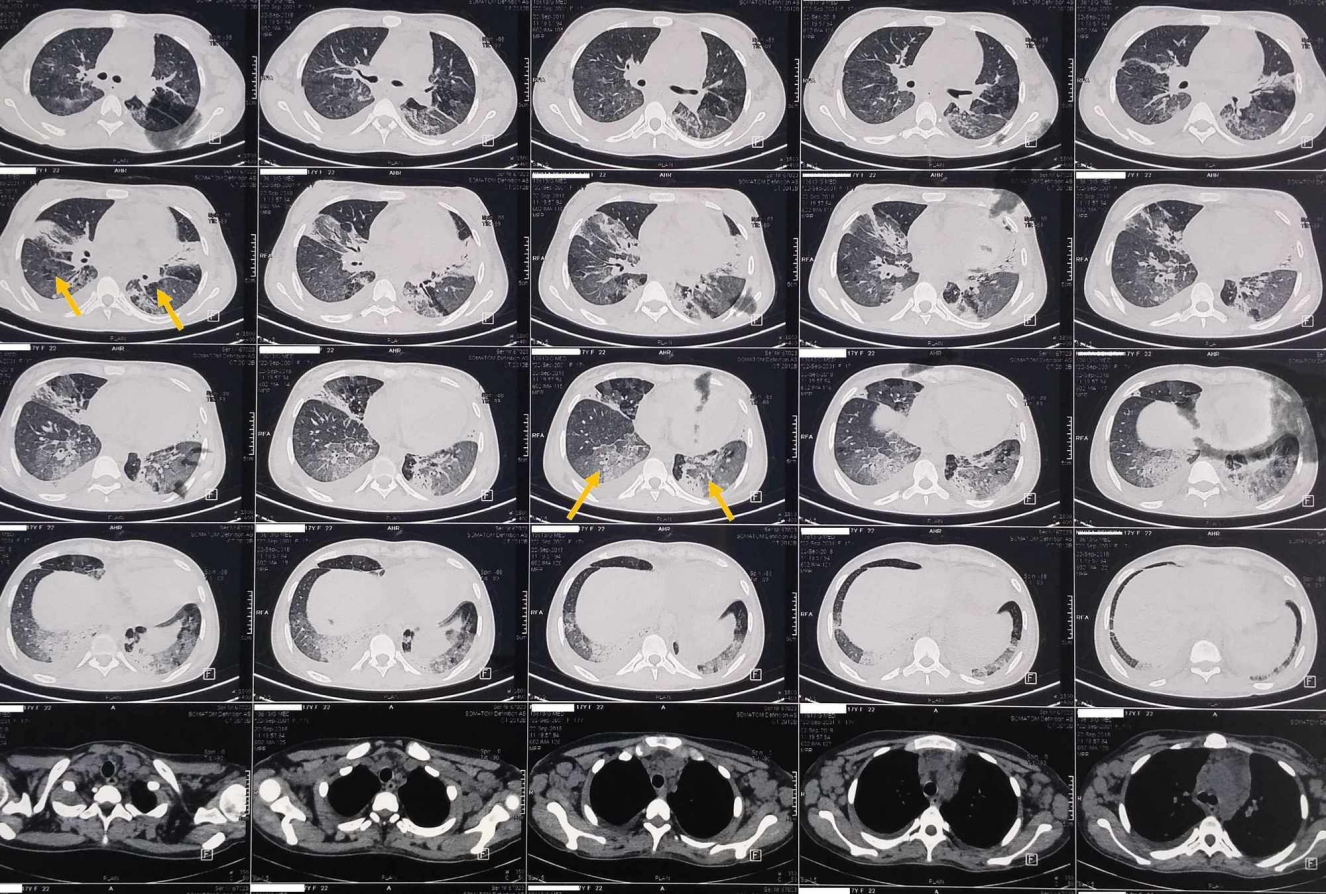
CT thorax showing bilateral ground-glass opacities
